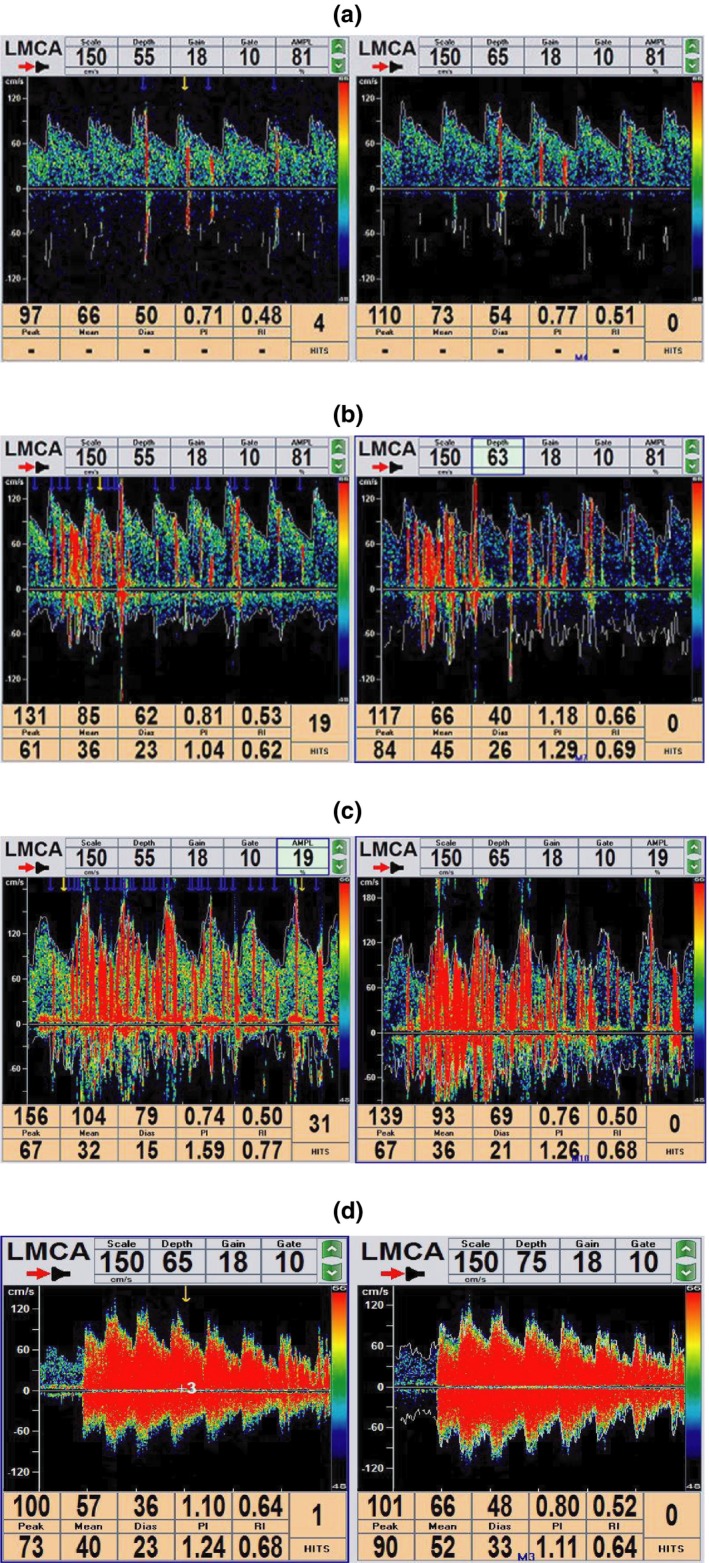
Three grades of shunt classification according to the microbubble (MB) counts in the Doppler spectrum *Note*. mild shunt = 1–10 MBs (a), moderate shunt =10–25 MBs (b), and large shunt= >25 MBs with shower (c) or curtain patterns (d)
